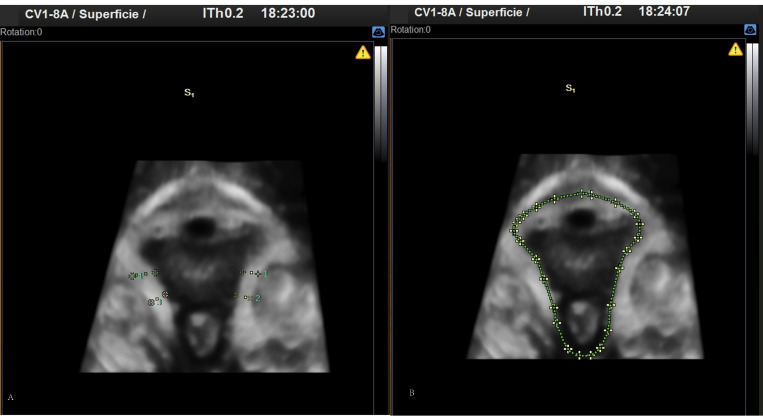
3D images obtained by perineal ultrasound. (A) Pubovisceral muscle thickness. (B) Perineal hiatus area.
